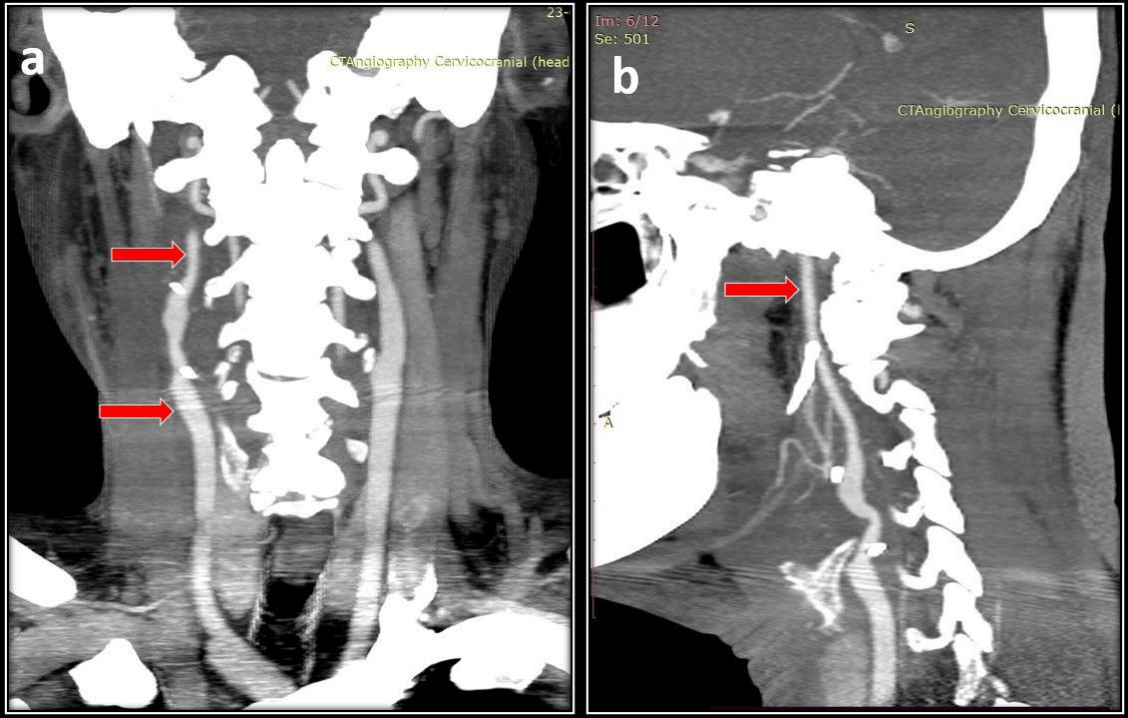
A) Postoperative follow-up CT angiography images showing a patent graft and B) patent circulation of the ICA.
